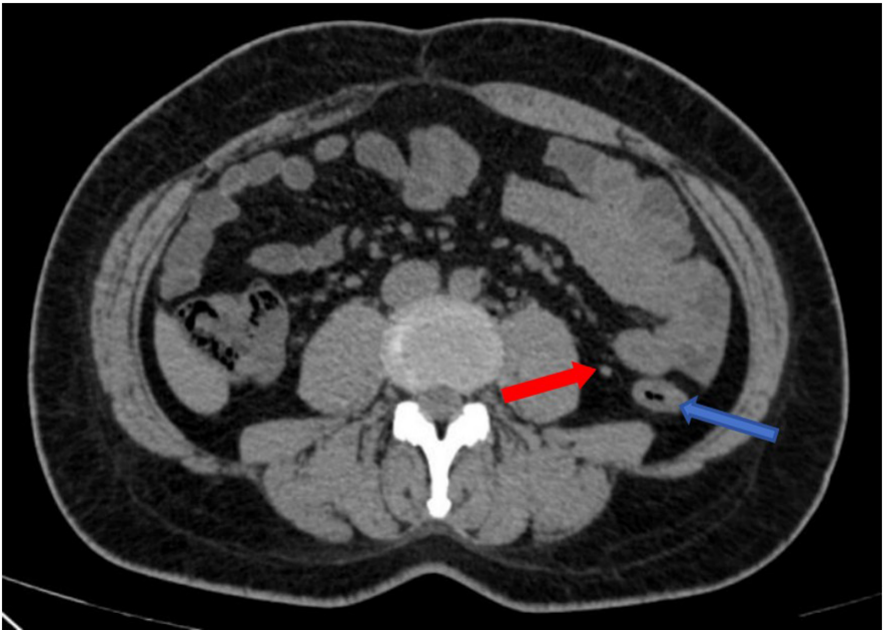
CT exam on May 24, 2024: Red arrow marks enlarged lymph nodes, and blue arrow marks the tumor location.
